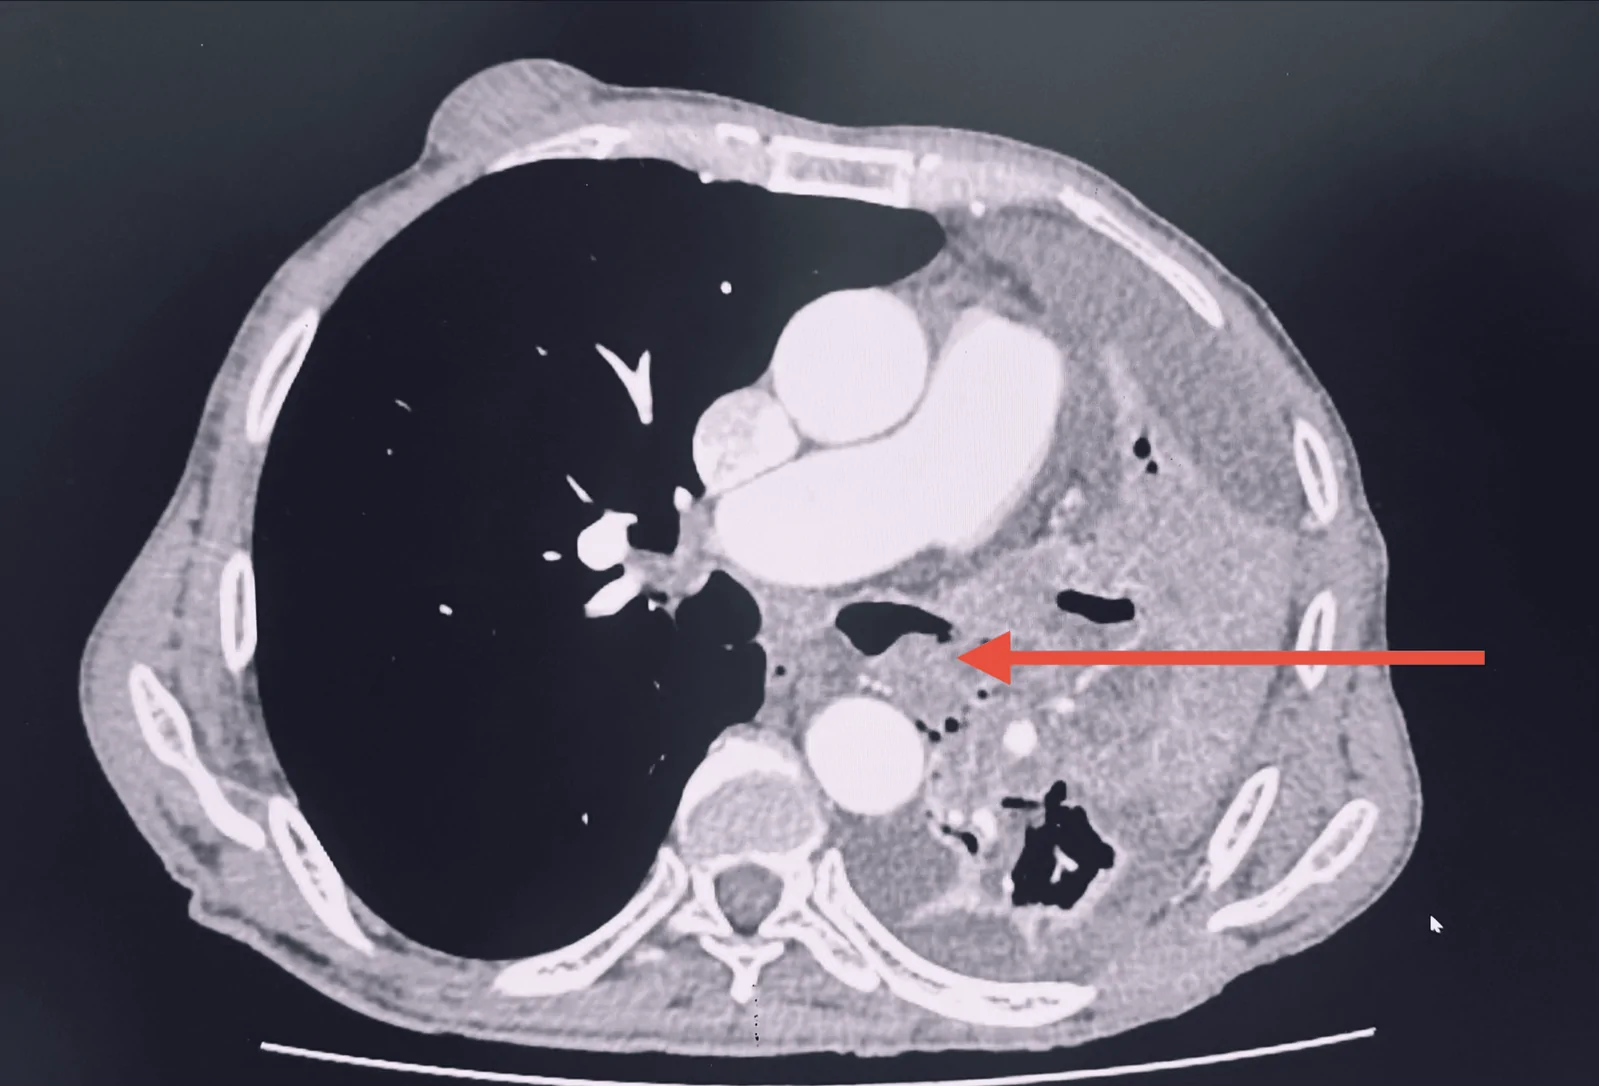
CECT of the chest demonstrating a 5.5*6.5*6.5 cm sized, heterogeneously enhancing, irregular, vague solid and cystic complex mass lesion in the left hilar region (red arrow) Contrast-enhanced computed tomography (CECT) of the chest (axial section) demonstrating a left hilar region mass (red arrow), causing complete obstruction of the left main bronchus and left lung collapse, and compressing the segmental branches of the left pulmonary artery with regional infiltration into mediastinal pleura.
